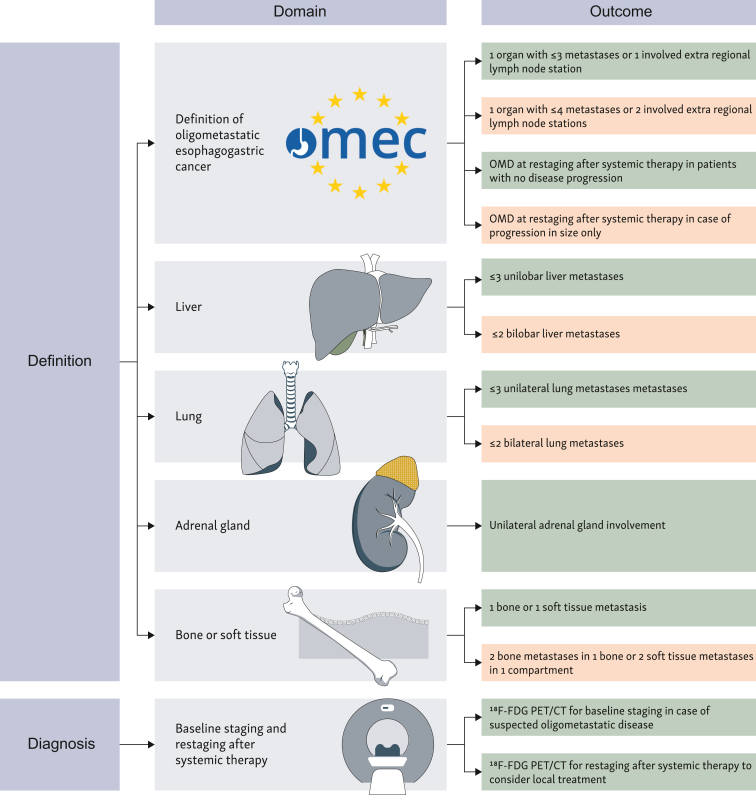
Definition and diagnosis of oligometastatic esophagogastric cancer according to the OMEC project. Green boxes indicating consensus (i.e. ≥75 agreement), orange boxes indicating fair agreement (50%-75% agreement). ^18^F-FDG PET–CT, [^18^F]2-fluoro-2-deoxy-D-glucose positron emission tomography–computed tomography; OMD, oligometastatic disease; OMEC, OligoMetastatic Esophagogastric Cancer.
